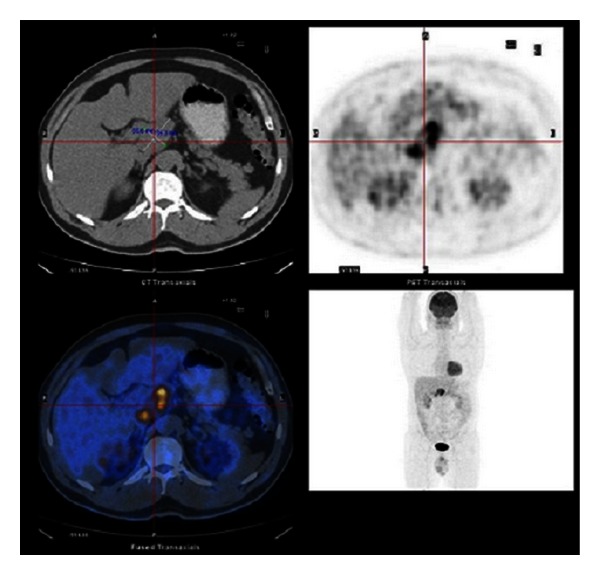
Axial and coronary images of ^18^F-FDG CT-PET. Upper and low left pictures represent plane CT and PET fusion image, respectively. Metabolically active lesion (yellow-red) located in the upper abdomen below the liver with high avidity to ^18^F-FDG on CT-PET fusion image (lower left).
